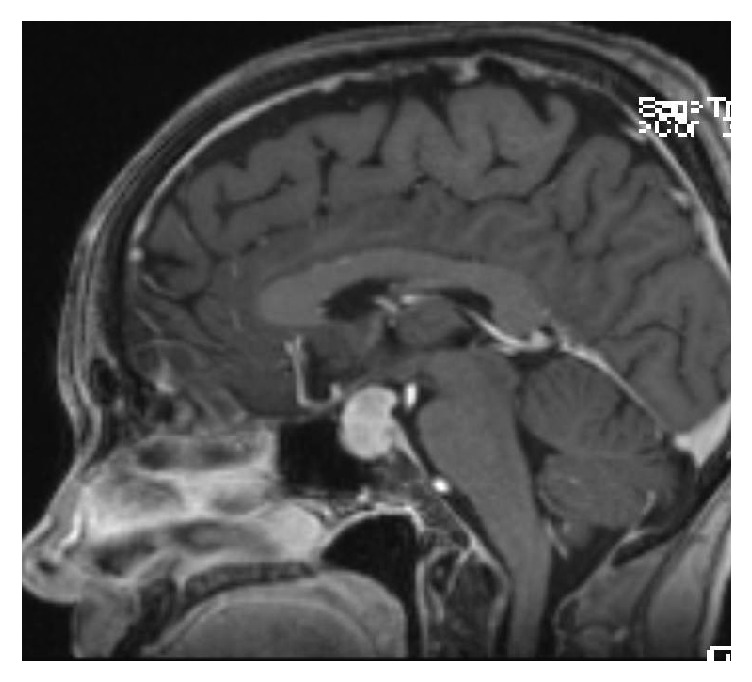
Preoperative sagittal MRI with contrast demonstrating the homogenously contrast enhancing sellar/suprasellar mass with mass effect on the optic chiasm.
